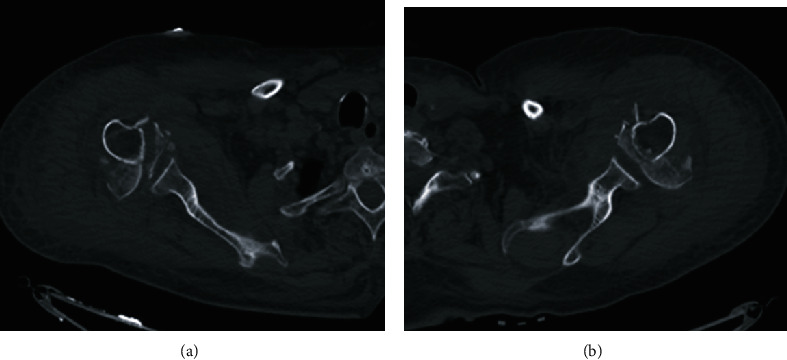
(a) CT of the right shoulder showing a comminuted displaced fracture of the proximal humerus. (b) CT of the left shoulder showing a comminuted displaced fracture of the proximal humerus.
